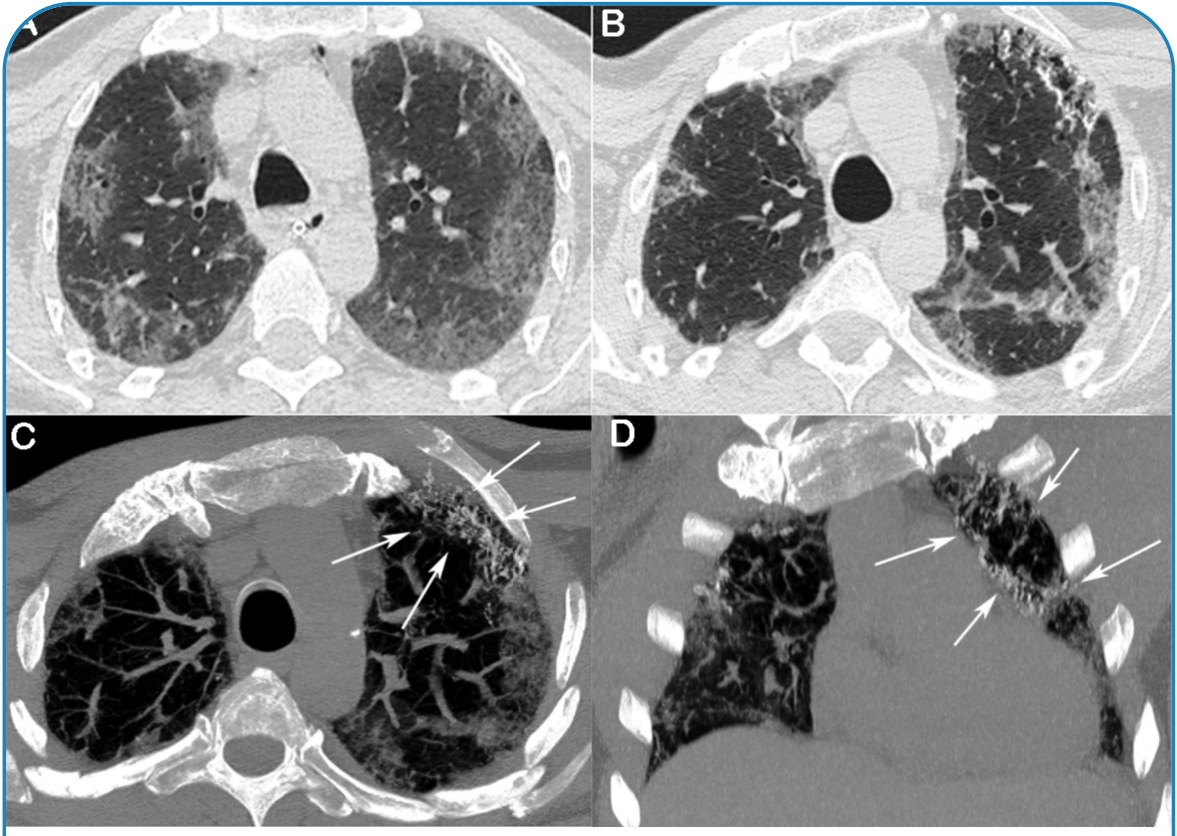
Axial computed tomography (CT) performed with lung window settings showed bilateral ground-glass opacities with mild reticulation (A). CT performed two months later demonstrated improvement of the ground-glass opacities but progression of the reticular opacities, with traction bronchiectasis on the anterior aspect of the superior lobes (B). Axial and coronal reconstructions acquired with mediastinal window settings showed interstitial calcifications compatible with dendriform pulmonary ossification over the reticular pattern (C and D,arrows).
